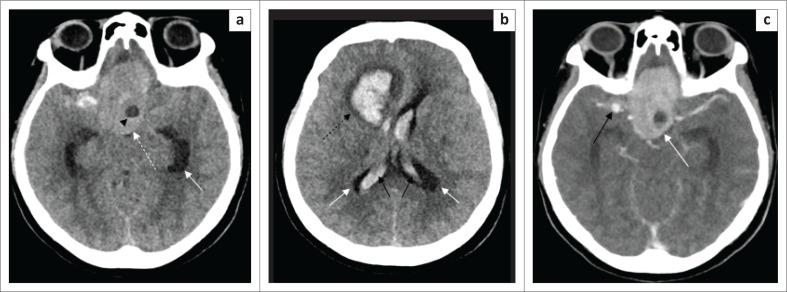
(a, b) Non-contrast CT axial images at the suprasellar cistern (a) and lateral ventricles (b) demonstrating a heterogeneous sellar-suprasellar mass (white dashed arrow) with cystic components and acute intralesional haemorrhage (arrowhead). Note the right frontal parenchymal haemorrhage (dashed black arrow) adjacent to the mass, intraventricular haemorrhage (black arrows) and obstructive hydrocephalus (white arrows). (c) Contrast-enhanced axial CT image demonstrating the enhancing sellar-suprasellar mass (white arrow) and a focal bright contrast collection in the right MCA cistern (black arrow), suspicious for a saccular aneurysm.
